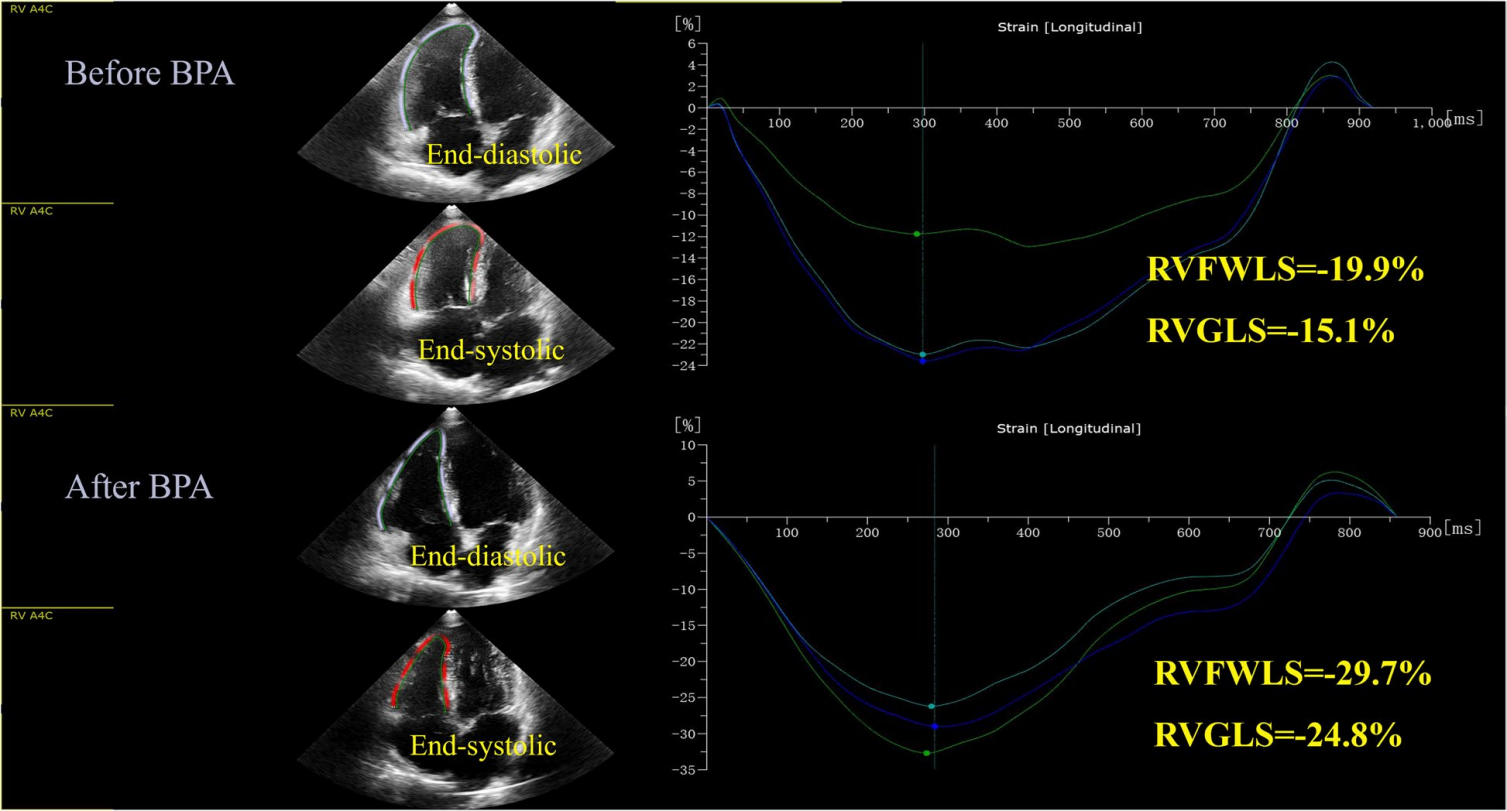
Right ventricular longitudinal strain measured by 2D-speckle tracking echocardiography (2D-STE) before and after balloon pulmonary angioplasty (BPA) in a patient. RVFWLS, RV free wall longitudinal strain; RVGLS, RV global longitudinal strain
